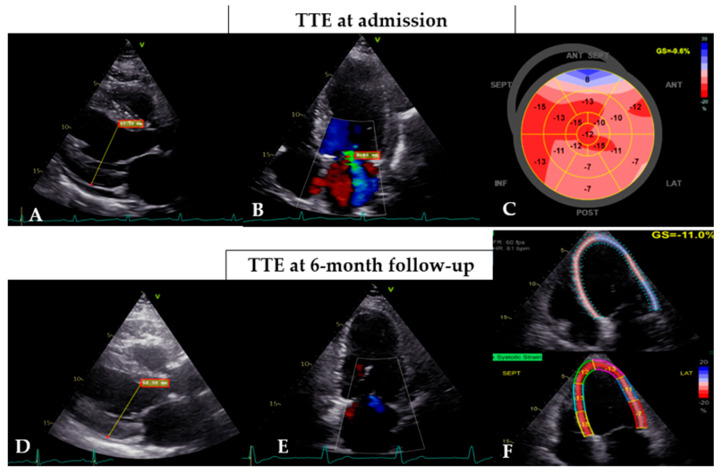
Baseline and 6-month follow-up TTE. Legend: TTE = transthoracic echocardiography. (A) Parasternal view with LV dimension measurement in diastole (63.18 mm); (B) apical 4-chamber view, moderate mitral regurgitation with a vena contracta width of approximately 6 mm; (C) baseline global longitudinal strain (GLS) bull’s-eye; (D) parasternal view at 6 months with improved LV dimension; (E) apical 4-chamber view, mild mitral regurgitation; (F) follow-up strain evaluation showing partial recovery.
